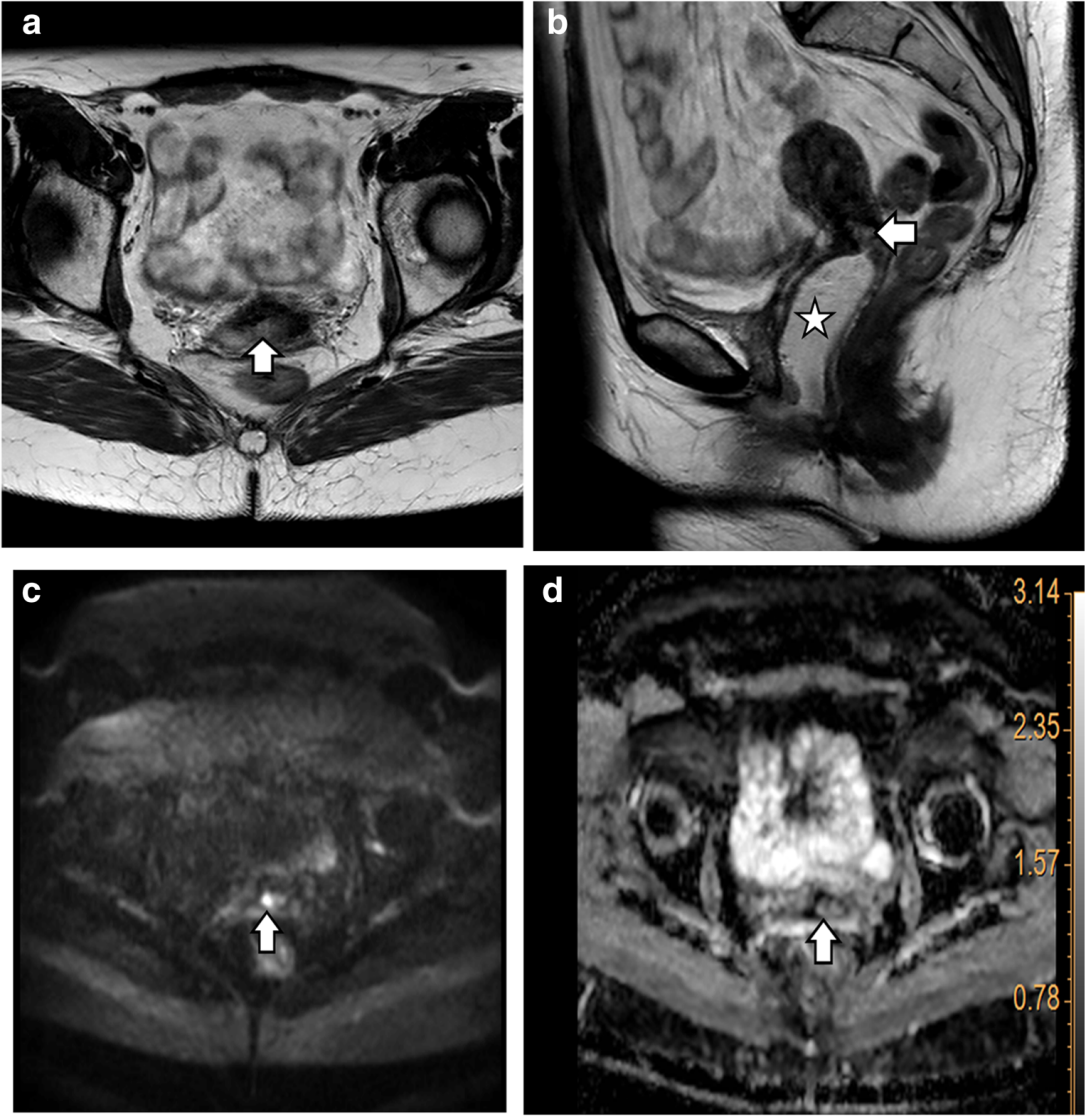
A 30-year-old patient with a normal post-therapeutic clinical examination. T2-weighted MR imaging (a, b arrow) showed an infracentimetric suspicious region centrally in the cervix. Local follow-up during 1 year remained normal. Diffusion-weighted imaging (DWI, c arrow) was not suspect for both readers. The ADC map (d arrow) was hyperintense (ADC, reader 1: 132 × 10^−4^ mm^2^/s and reader 2: 125 × 10^−4^ mm^2^/s), confirming the presence of fluid rather than tumour. The delta ADC was not suspect for readers (δADC, reader 1: 63 × 10^−4^ and reader 2: 52 × 10^−4^) on the basis of aforementioned cut-off of 31 × 10^−4^. Note the use of intravaginal gel in order to expand the vagina on b (white star)
